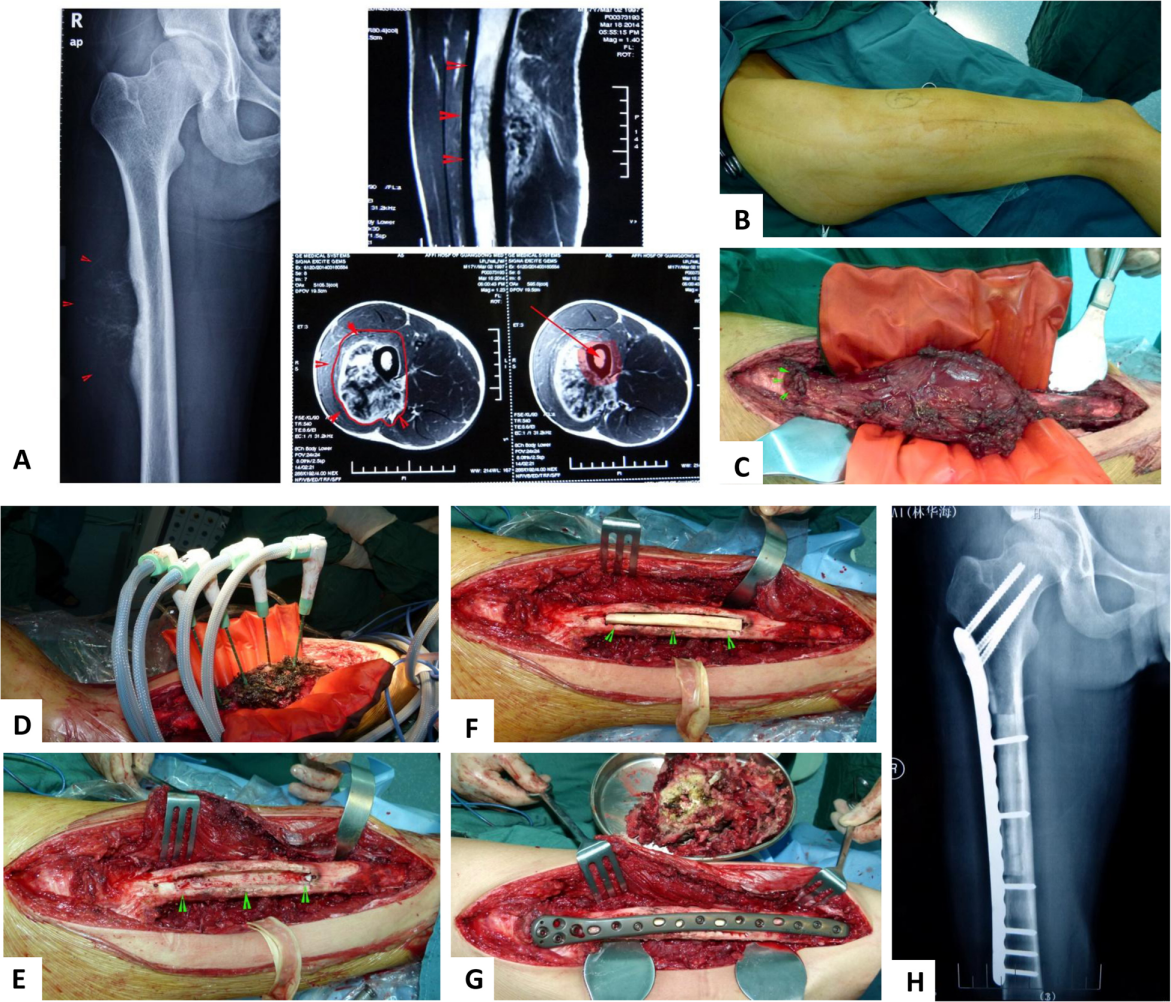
Typical procedure for malignant bone tumors at femoral diaphysis. a Image data of A-P X ray and MRI films demonstrated an osteosarcoma at femoral diaphysis before surgery. There were distinct signs of cortical bone destruction in the middle part of the right femoral shaft and visible soft tissue mass shadow in the anterolateral range of quadriceps femoris. b The incision demonstration from lateral thigh after skin degerming. c Dissect the tumor-bearing bone from surrounding normal tissues with safe margin and a heat-isolation pad was put between the tumor bone and surrounding normal tissues. d The microwave generator and antenna were inserted into the tumor bulk and began to deliver electromagnetic energy into the tumor bone. e Soft tumor mass and tumor bone inside the femoral diaphysis were removed and bone graft cavity was prepared. f The mixture materials of autologous fibular graft with allograft bone chips or bone cement were used for filling the cavity. g Restore the normal shape of the femoral diaphysis and give a prophylactic fixation. h Postoperative A-P X ray film showed the complete tumor removal and perfect bone transplantation
